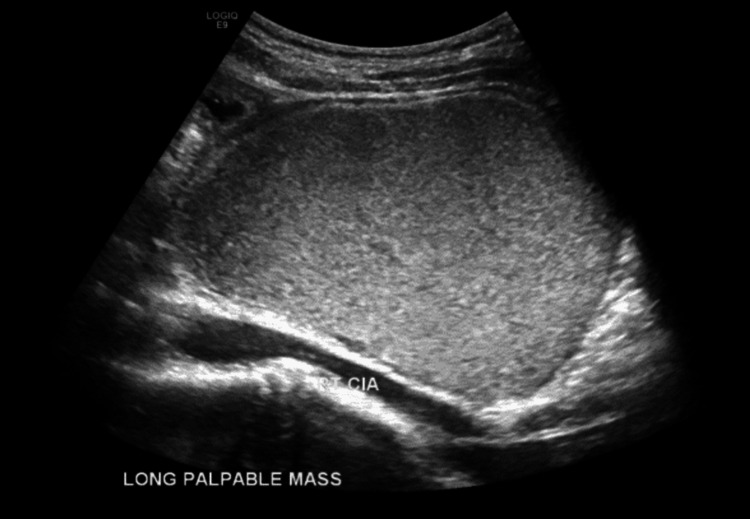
Ultrasound image of a complex mesenteric or ovarian cyst or a solid lesion such as desmoid tumor
